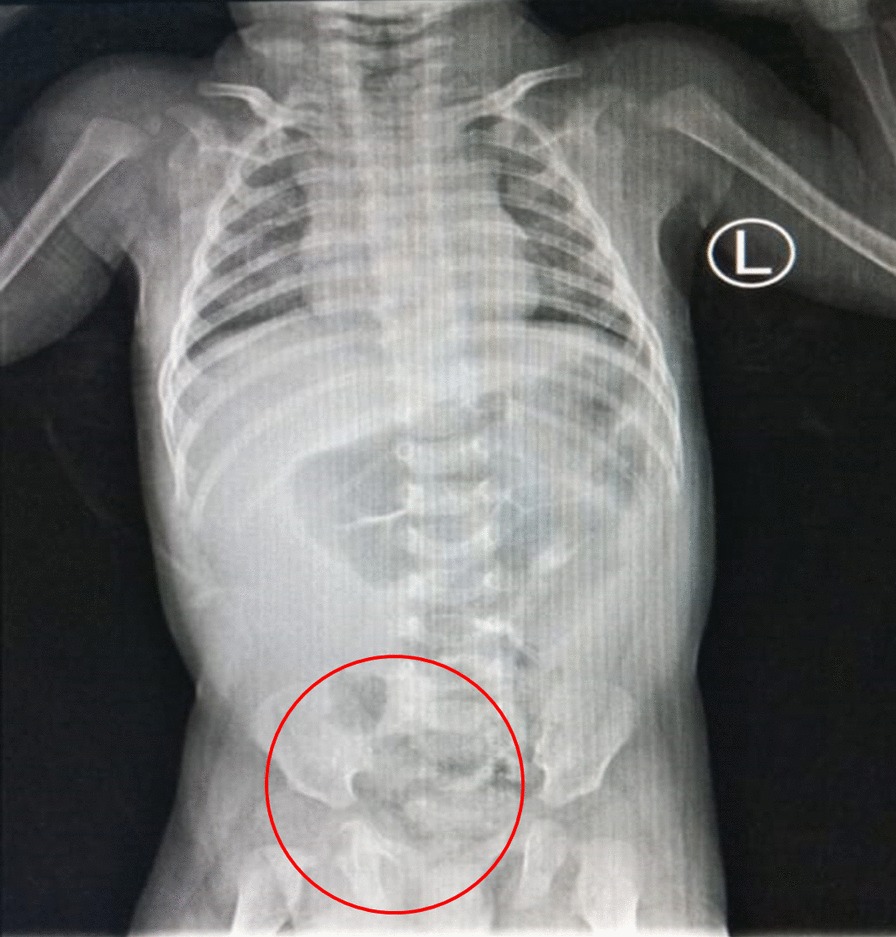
Plain abdominal radiography
